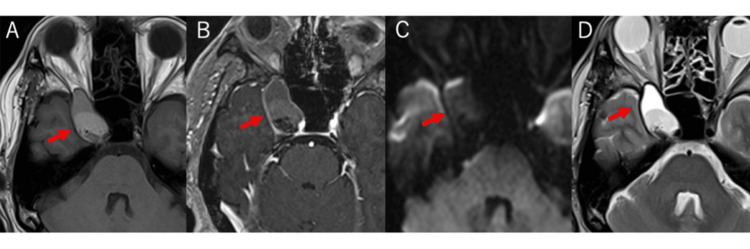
MRI images. A: T1-weighted image; B: Gadolinium-diethylenetriaminepentaacetate (Gd-DTPA) image; C: Diffusion-weighted image; D: T2-weighted image. MRI showed a multiloculated cyst containing fluid of mixed intensity, with no solid contrast-enhancing component.
